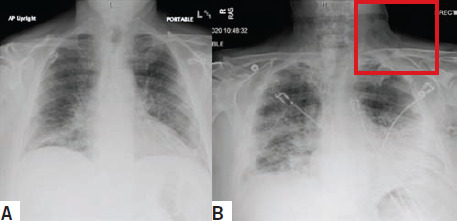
Admission chest radiograph (A) showing bilateral infiltrates. Chest radiograph on hospital day 21, revealing subcutaneous emphysema and pneumothorax at the left lung apex (B, box).
